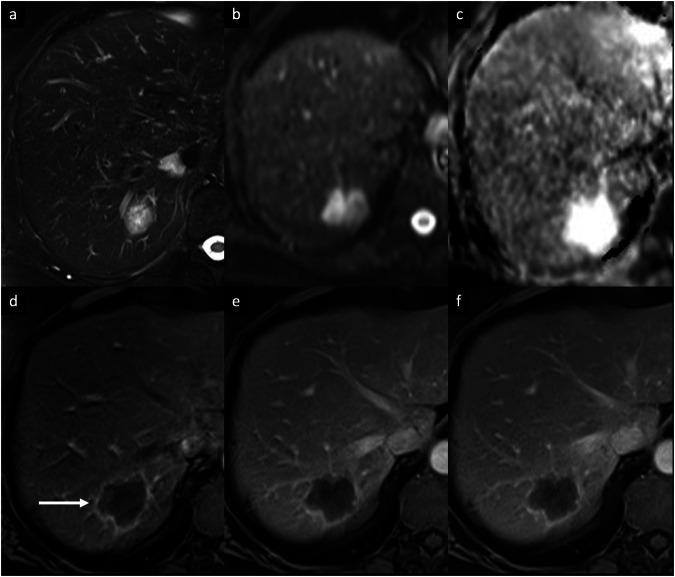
Sclerosed hemangioma in a 71-year-old woman who presented with abdominal pain and inflammatory syndrome. a Axial T2-weighted MRI shows a focal liver lesion in the right lobe with increased SI, with a hyperintense signal on diffusion-weighted imaging (b) but with high intensity on the ADC map (c). Extracellular contrast agent-enhanced MRI demonstrates a rim APHE (arrow) (d) that persisted and minimally increased on portal venous (e) and delayed phases (f). A biopsy of the lesion confirmed the diagnosis of sclerosed hemangioma
